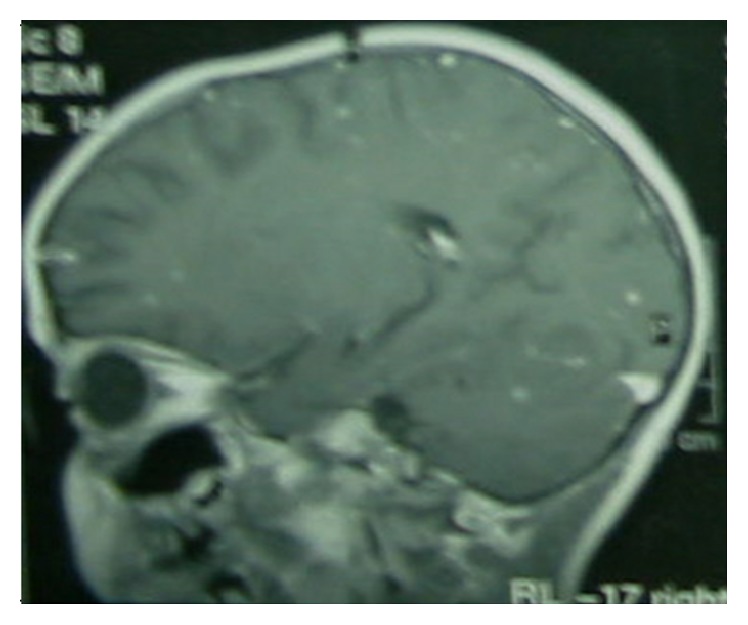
Tuberculomas on cranial MRI.
